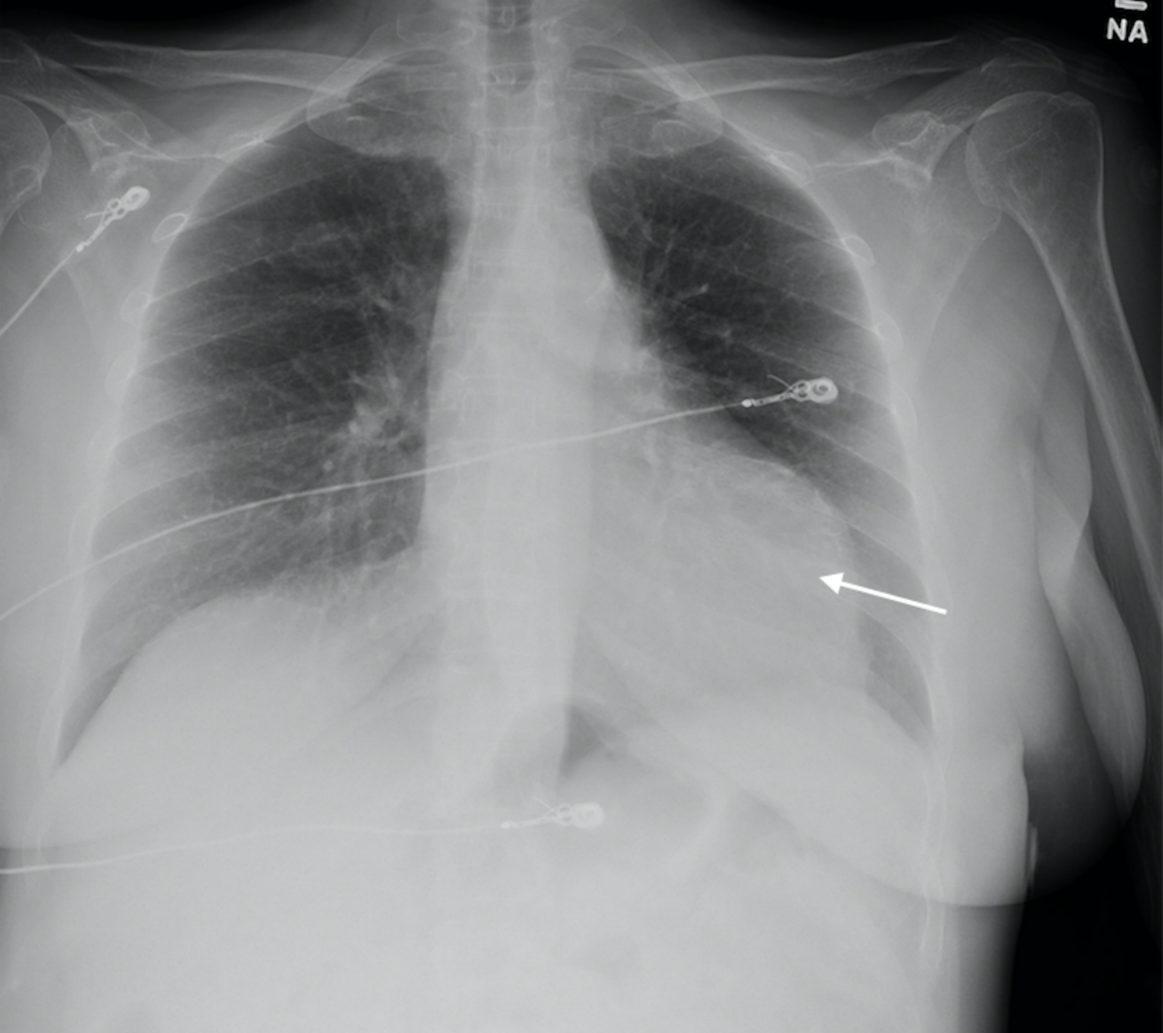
Chest radiograph demonstrated abnormal heart contour suggestive of aneurysmal dilatation (arrow).
